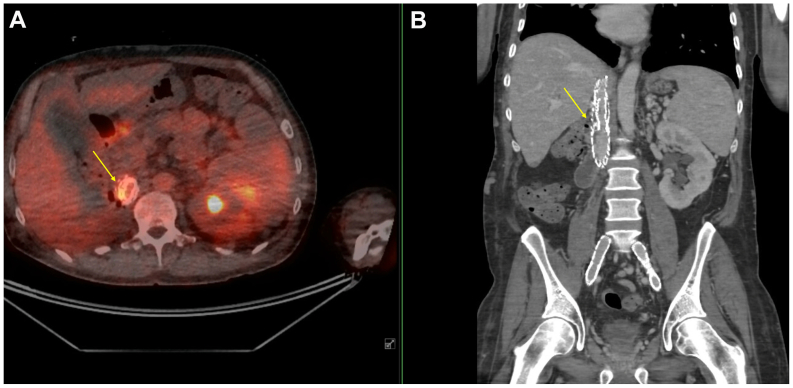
Positron emission tomography-computed tomography (PET-CT) shows hypermetabolism on the stent adjacent to the duodenum. A, Transversal PET-CT image. B, Coronal CT image. The *yellow arrow* indicates the infected stent.
